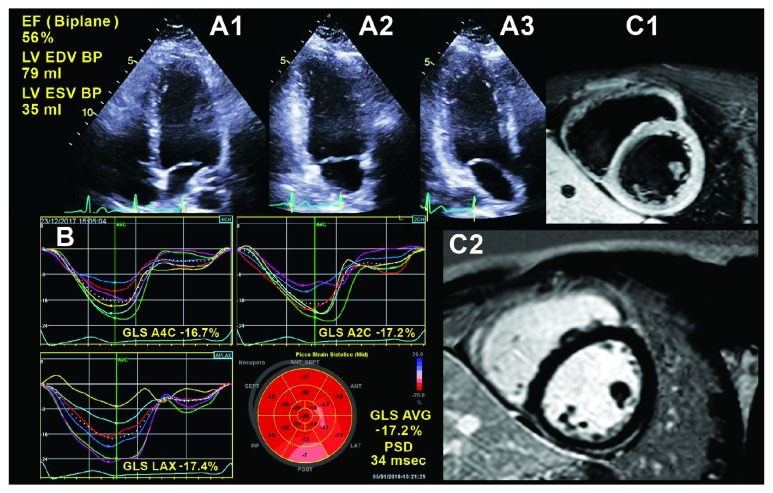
A 27-year-old male patient with myocarditis. Panel A1-3 shows normal left ventricular systolic function assessed by Simpson's method. Panel B shows left ventricular two-dimensional speckle-tracking echocardiography longitudinal strain curves and bull's-eye map: please note that the bull's-eye plot underlines regional alterations of longitudinal function in the inferolateral wall. In Panel C1-2, cardiac magnetic resonance shows a hyperenhancement area located in the epicardial layer of the inferior and inferolateral left ventricular walls, suggesting edema and fibrosis, respectively, on both T2 weighted and late gadolinium enhancement images; please note that bull's-eye data from two-dimensional speckle-tracking echocardiography are confirmed by the assessment of fibrosis in the same segments by cardiac magnetic resonance.
